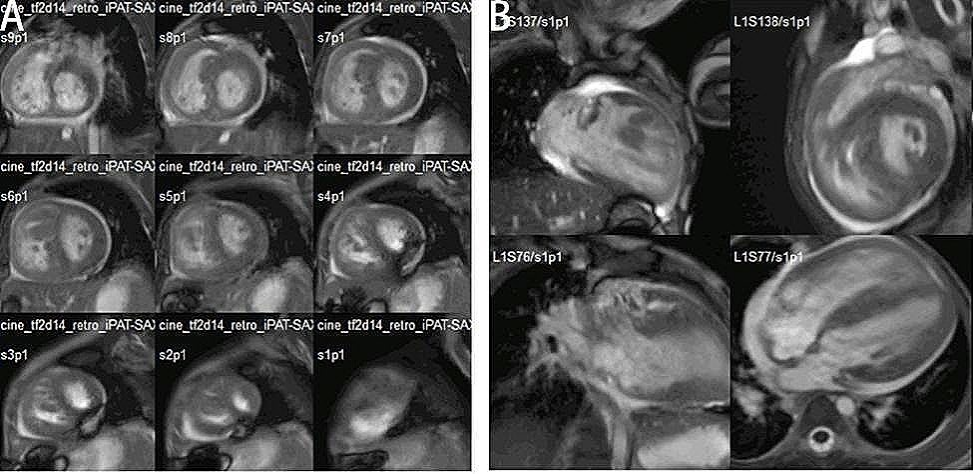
Cardiac MRI: ventricular hypertrophy
